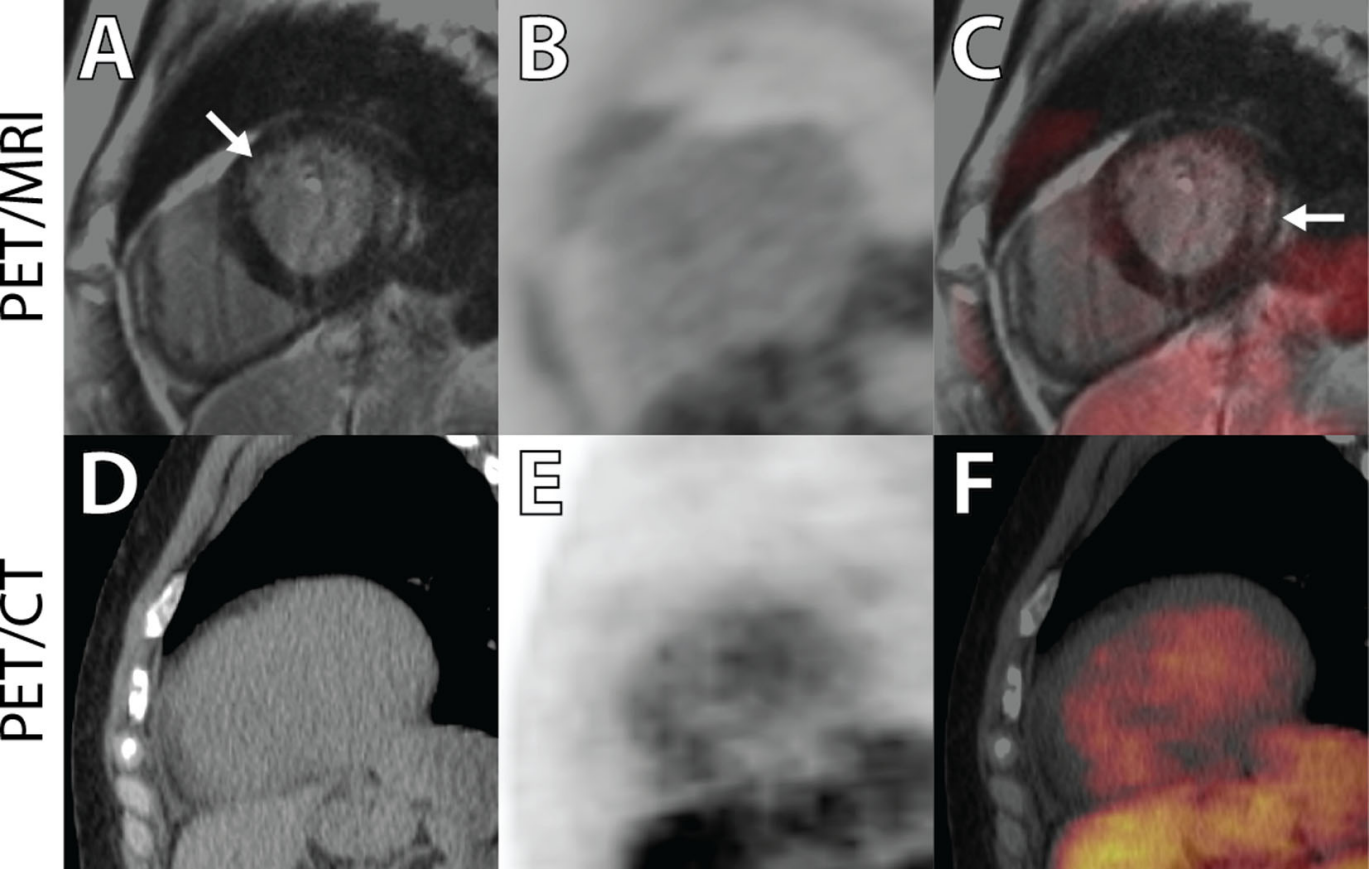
Images from patient three. In this case, an enhanced signal is seen on the delayed enhancement MR images in both mid-septum and lateral regions (A) [arrows]. There is no corresponding increase in ^18^F-FDG signal on the PET images (B, C). Note the increased blood-pool activity on the PET/CT images that were taken approximately 2 h earlier (see Table 4), from the same single injection of ^18^F-FDG (E, F). The ejection fraction for this patient was 52% with mild inferoseptal hypokinesis
